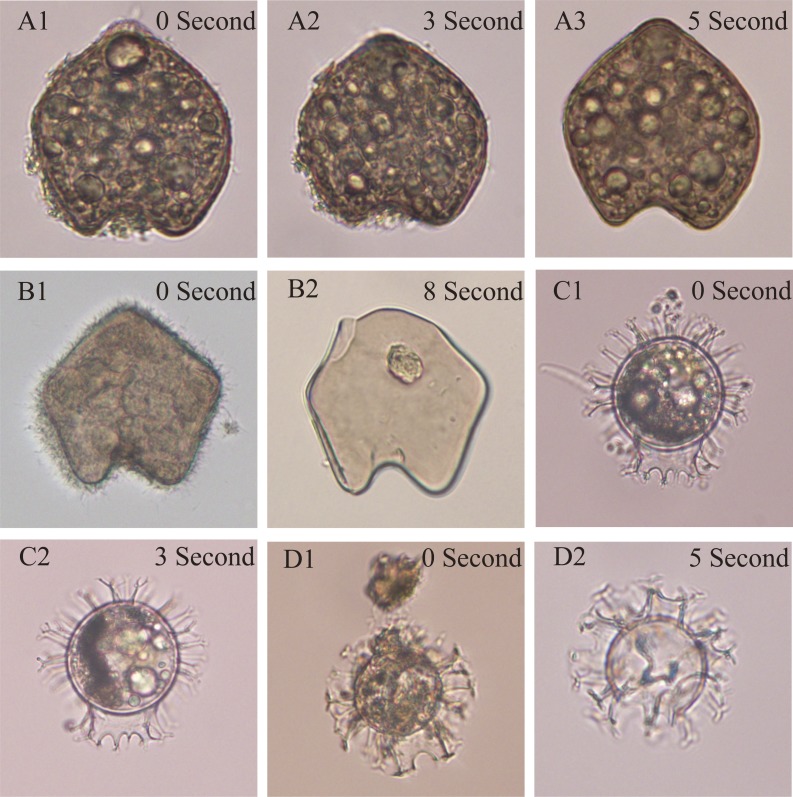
The test of optimized ultrasonic cleaning time on two typical species belonging to two major groups (*Protoperidinium* and *Gouyaulax*). A1-3 and B1-2, and C1-2 and D1-2 show the same species: * Protoperidinium oblongum* and *Gouyaulax spinifera*, respectively, but were treated with different ultrasonic cleaning times.
